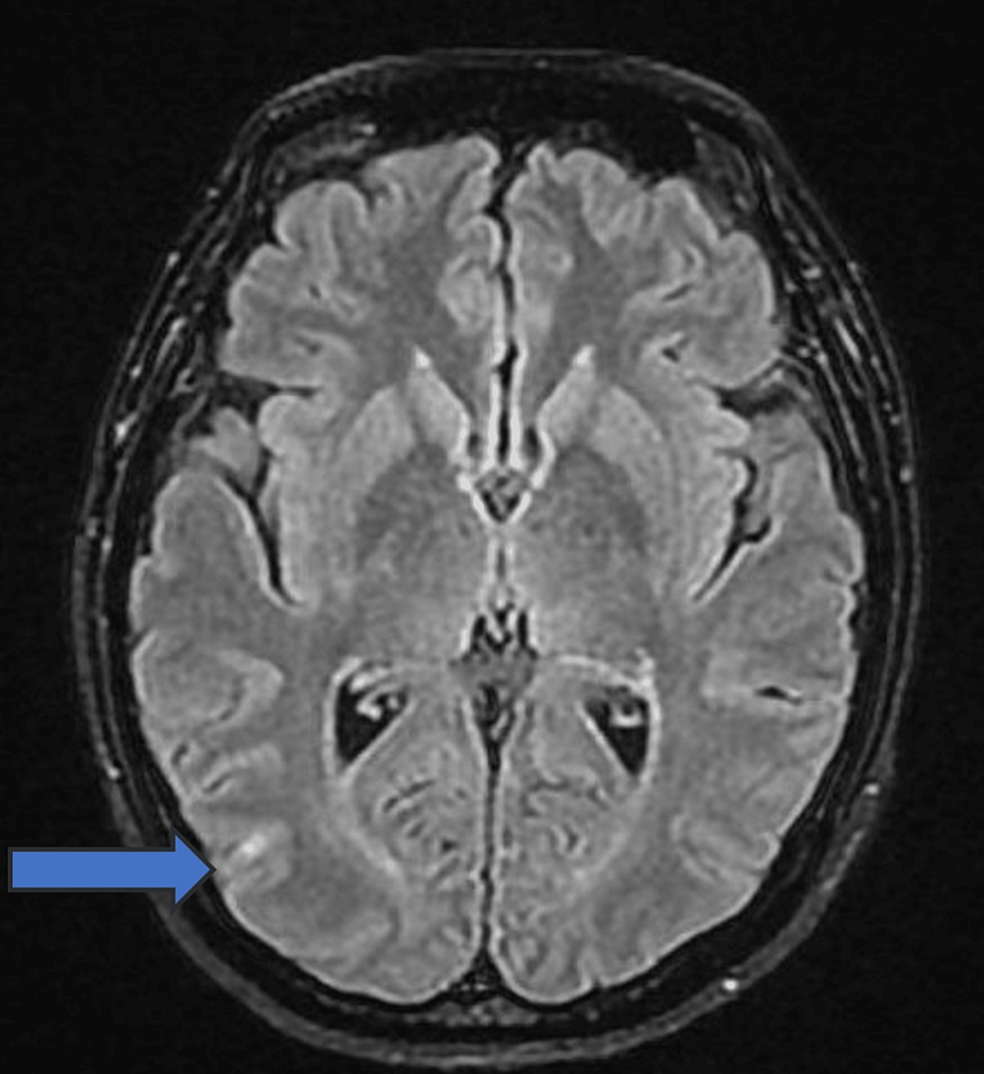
Brain MRI (T2) Note the hypersignal in the temporo-parieto-occipital junction (TPO)
